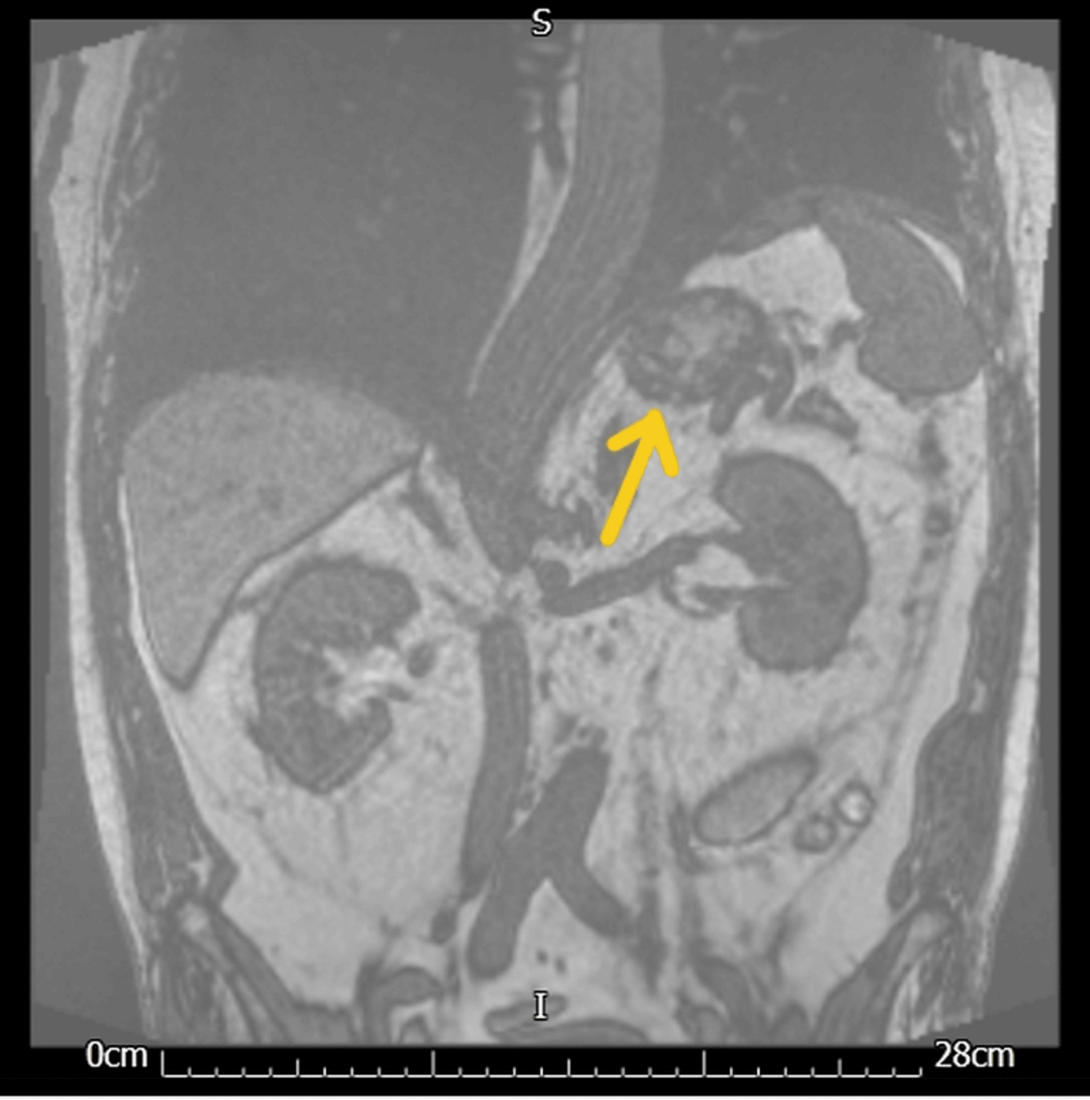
MRI adrenal glands following the CT pulmonary angiogram (CTPA) MRI adrenal gland (coronal T1-weighted phase 1): the yellow arrow shows the lesion involving the left adrenal gland identified, which measured 32 x 31 mm and showed no significant interval change in comparison to a previous study.
